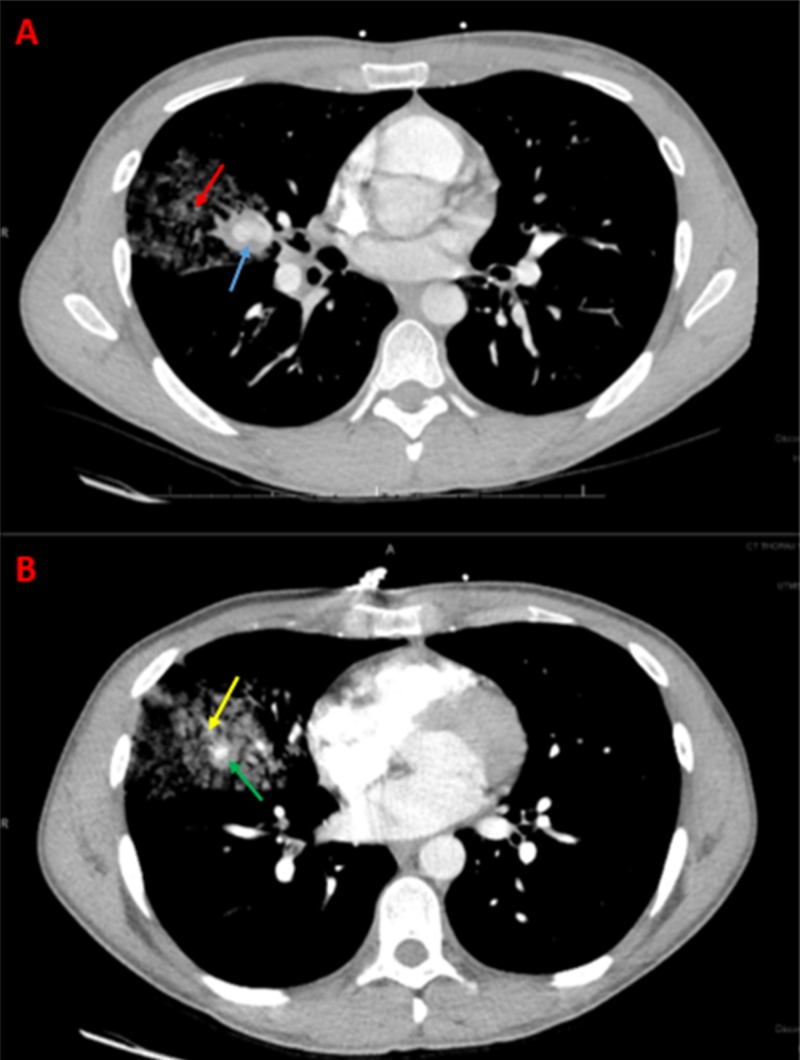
Axial Computed Tomography Angiogram Demonstrating Pulmonary Artery Aneurysms and Respective Hemorrhage An axial computed tomography angiogram of the chest demonstrates two separate pulmonary artery aneurysms in the right middle lobe associated with a surrounding pulmonary artery hemorrhage. The top frame (A) shows the most proximal aneurysm (blue arrow) with the respective hemorrhage (red arrow). The bottom frame (B) shows a more distal aneurysm (green arrow) with the respective hemorrhage (yellow arrow).
